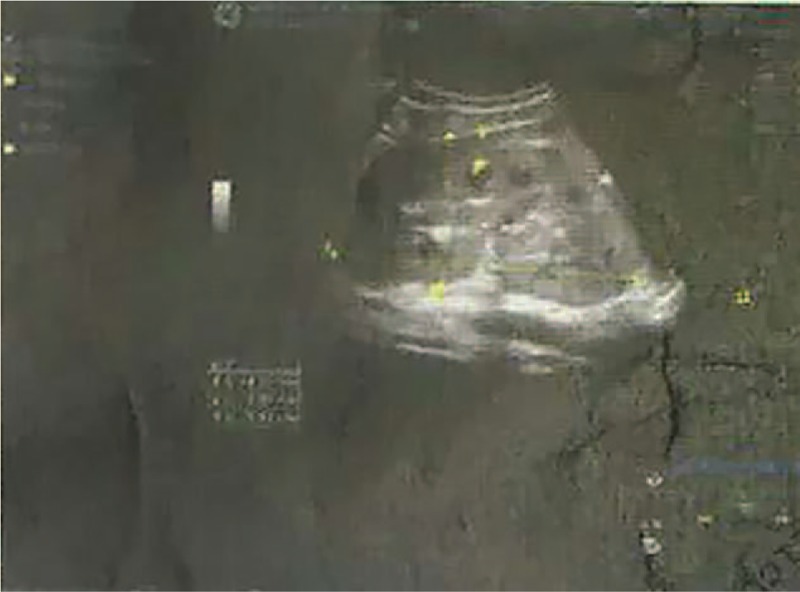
Abdominal ultrasonography revealed symmetrical homogenous kidneys with hyperechogenic pattern and poor corticomedullary differentiation.
